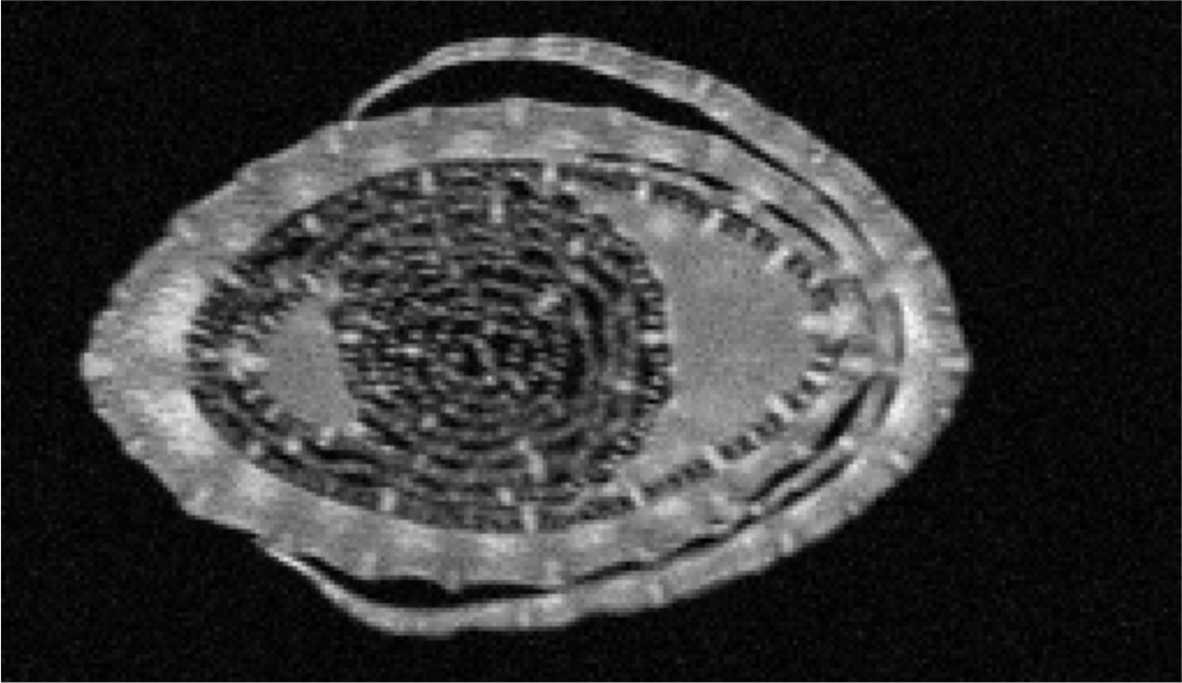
MRI scan through the pseudostem of a maize seedling. B104 seedlings were scanned at ca 5 cm from the base of the plant, three days after emergence of the 5^th^ leaf. The outer most leaf in the image is the second leaf, which is surrounding the third. Both are mature at this stage. The growing 4^th^ and 5^th^ leaves are curled up inside. Scale: Full image represents an area of 10x6mm.
